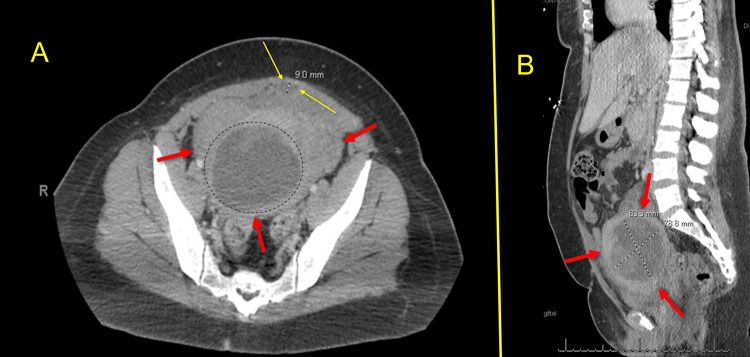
CT scan of the pelvis (transverse, image A) and abdominal/pelvic (sagittal, image B) (day 46 after initial admission and 30 days after cesarean delivery). The long yellow arrows in image A show a near resolution of the midline rim-enhancing fluid collection measuring less than 1 cm. Also, within the black hashed circle, there is a mild increase noted in the “cystic lesion within the uterus” (8.8 cm x 7.8 cm x 8.1 cm), suggestive of a necrosed fibroid versus retained products versus a uterine abscess. The uterine wall is indicated by the red arrows. Image B shows the mass within the uterus (hash marks), with the red arrows showing the uterine wall.
